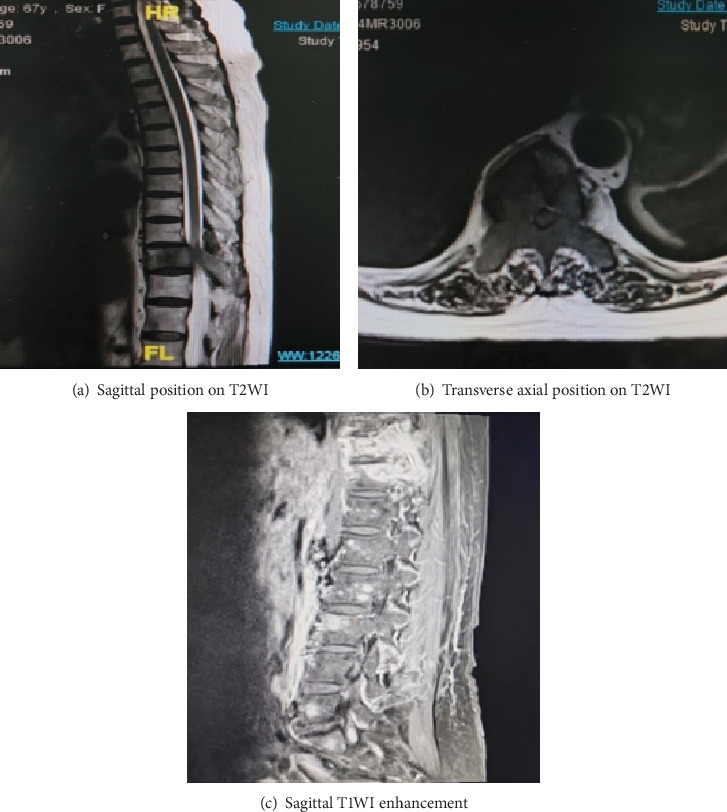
MRI imaging from June 2022. (a) Sagittal position on T2WI indicated decreased T10 vertebral signal, mild deformation, poor T9/10 alignment, local spinal stenosis, and acceptable upper and lower intervertebral discs. (b) Transverse position on T2WI indicated diffuse reduction of signals in the T10 vertebrae and annexes and slight swelling of local bone structure. (c) Sagittal T1WI enhancement indicated multiple lamellar enhancement foci in thoracolumbar vertebrae and adnexa, with a focus on the T10–11 vertebrae.
